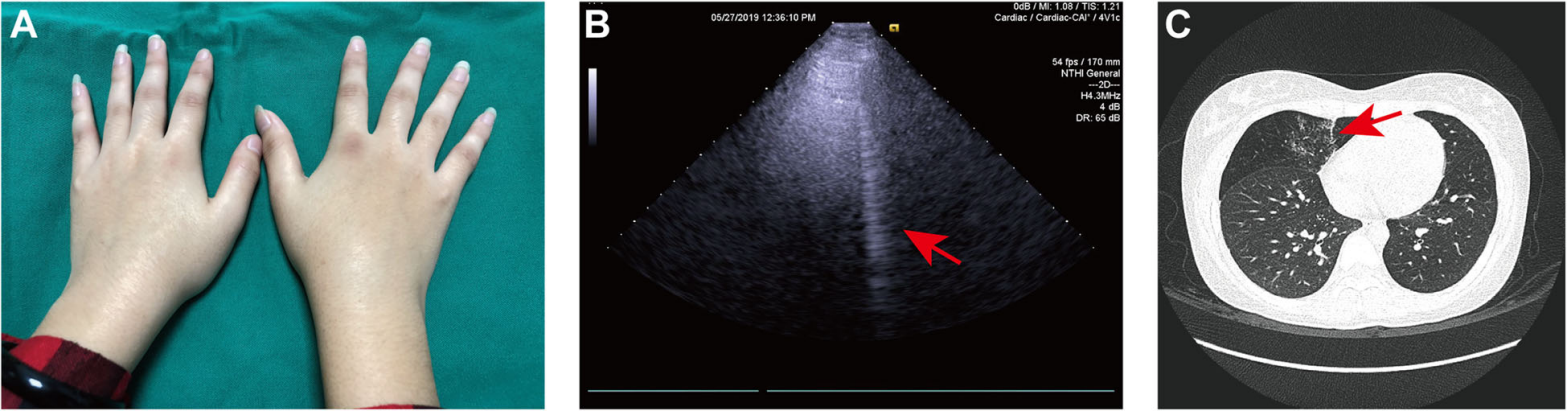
Case 2. A Physical examination showed polyarthritis of the hand joint. B Lung ultrasound revealed B-lines (arrow). C HRCT demonstrated ground glass opacity and interlobular septal thickening pattern located at the anterior segment of the right upper lobe (arrow)
